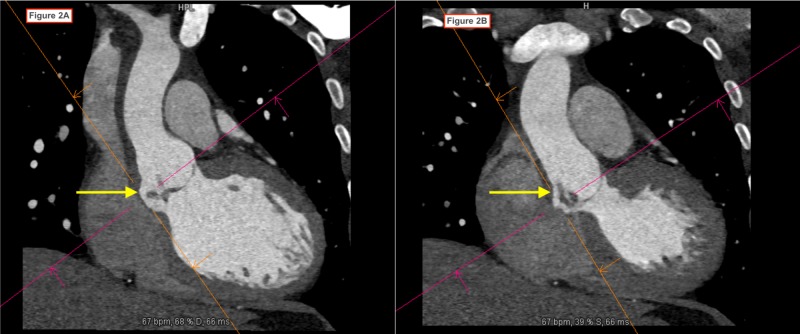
Cardiac CT scan showing aortic root pseudo-aneurysm involving the non-coronary sinus of Valsalva with a fistula from the aortic root to the left ventricle.
